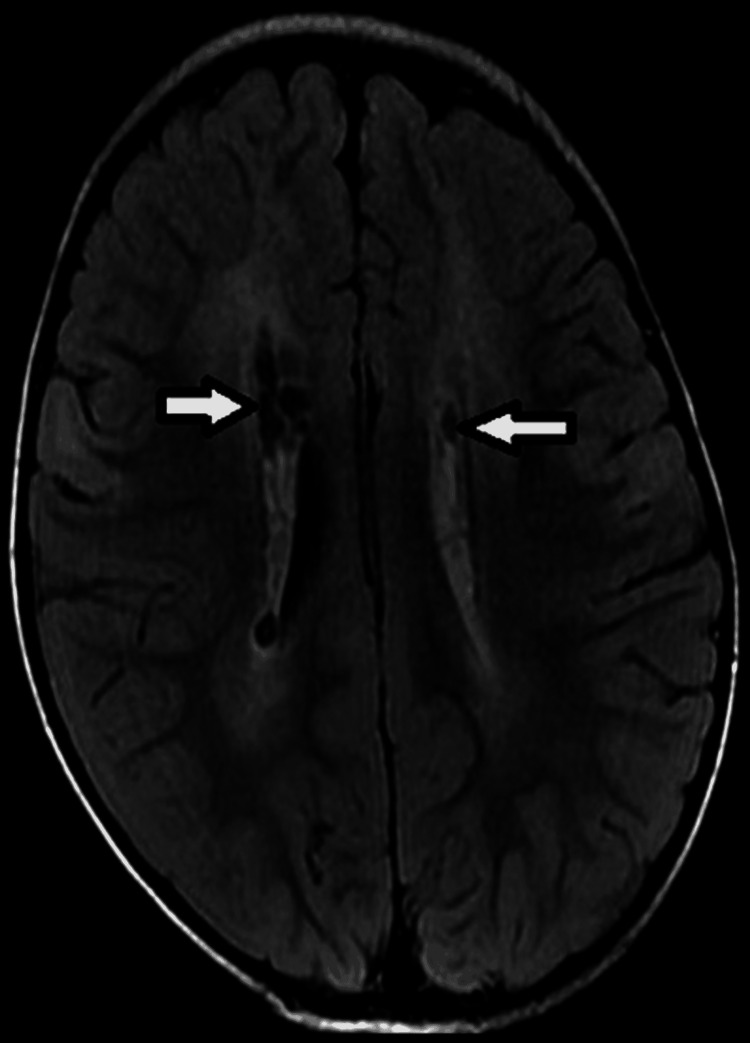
Axial T2 FLAIR images of a three-year-old child with developmental delay, at the level of the Corona radiate, showing periventricular cystic encephalomalacia and adjacent gliosis due to perinatal hypoxic insult (white arrows).
